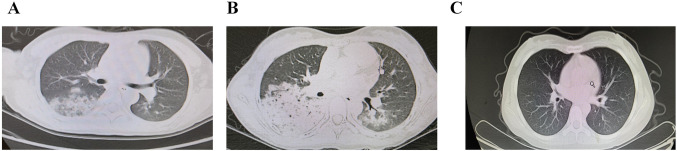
High-resolution computed tomography (HRCT) of bilateral alveolar infiltrates is suggestive of diffuse alveolar hemorrhage. HRCT of the chest showed multiple patches with increases in density and peripheral opacities in the 2nd hospital day (A). There was massive infiltrates in right lobes, accompanied by pleural effusions in the fourth hospital day, which demonstrated an obvious radiological progression (B). There was neither inflammatory exudation nor pleural effusion, as demonstrated by chest CT re-examination in the 4th month of the follow up (C)
